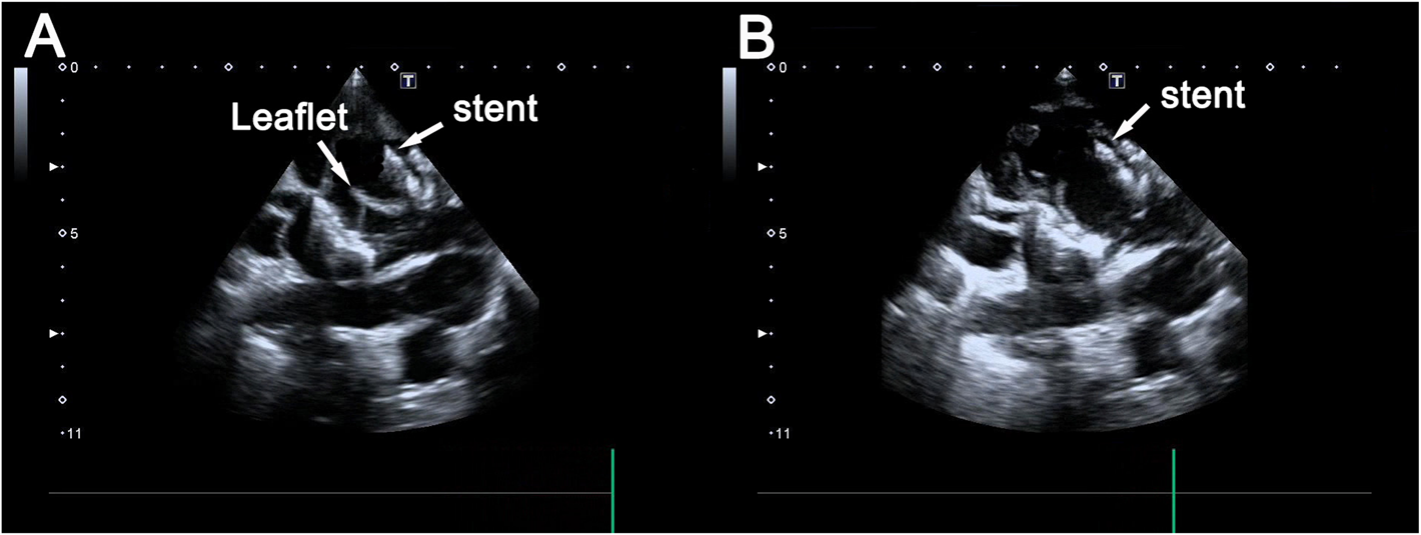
Epicardial echocardiography reveals excellent device function with complete leaflet closing (a) and opening (b)
